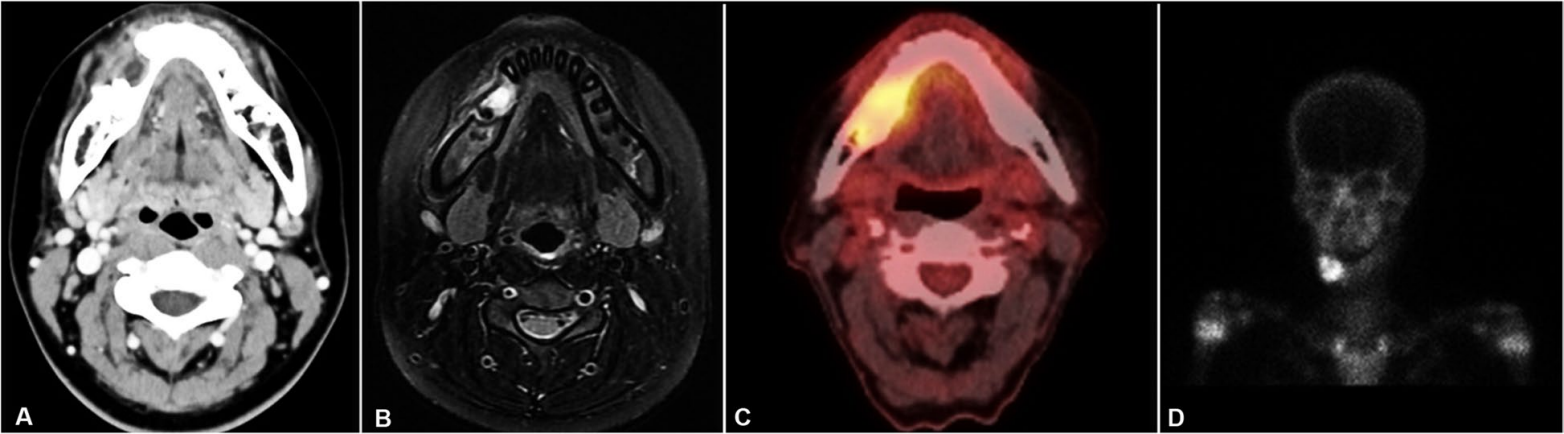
Pre-operative imaging. a Enhanced CT showing periosteal reaction at the buccal side. b MRI showing diffuse enhancement of soft tissue at the adjacent buccal area. c PET-CT showing a soft tissue lesion at the right premolar lesion. d Bone scintigraphy showing increased uptake in the right mandible
